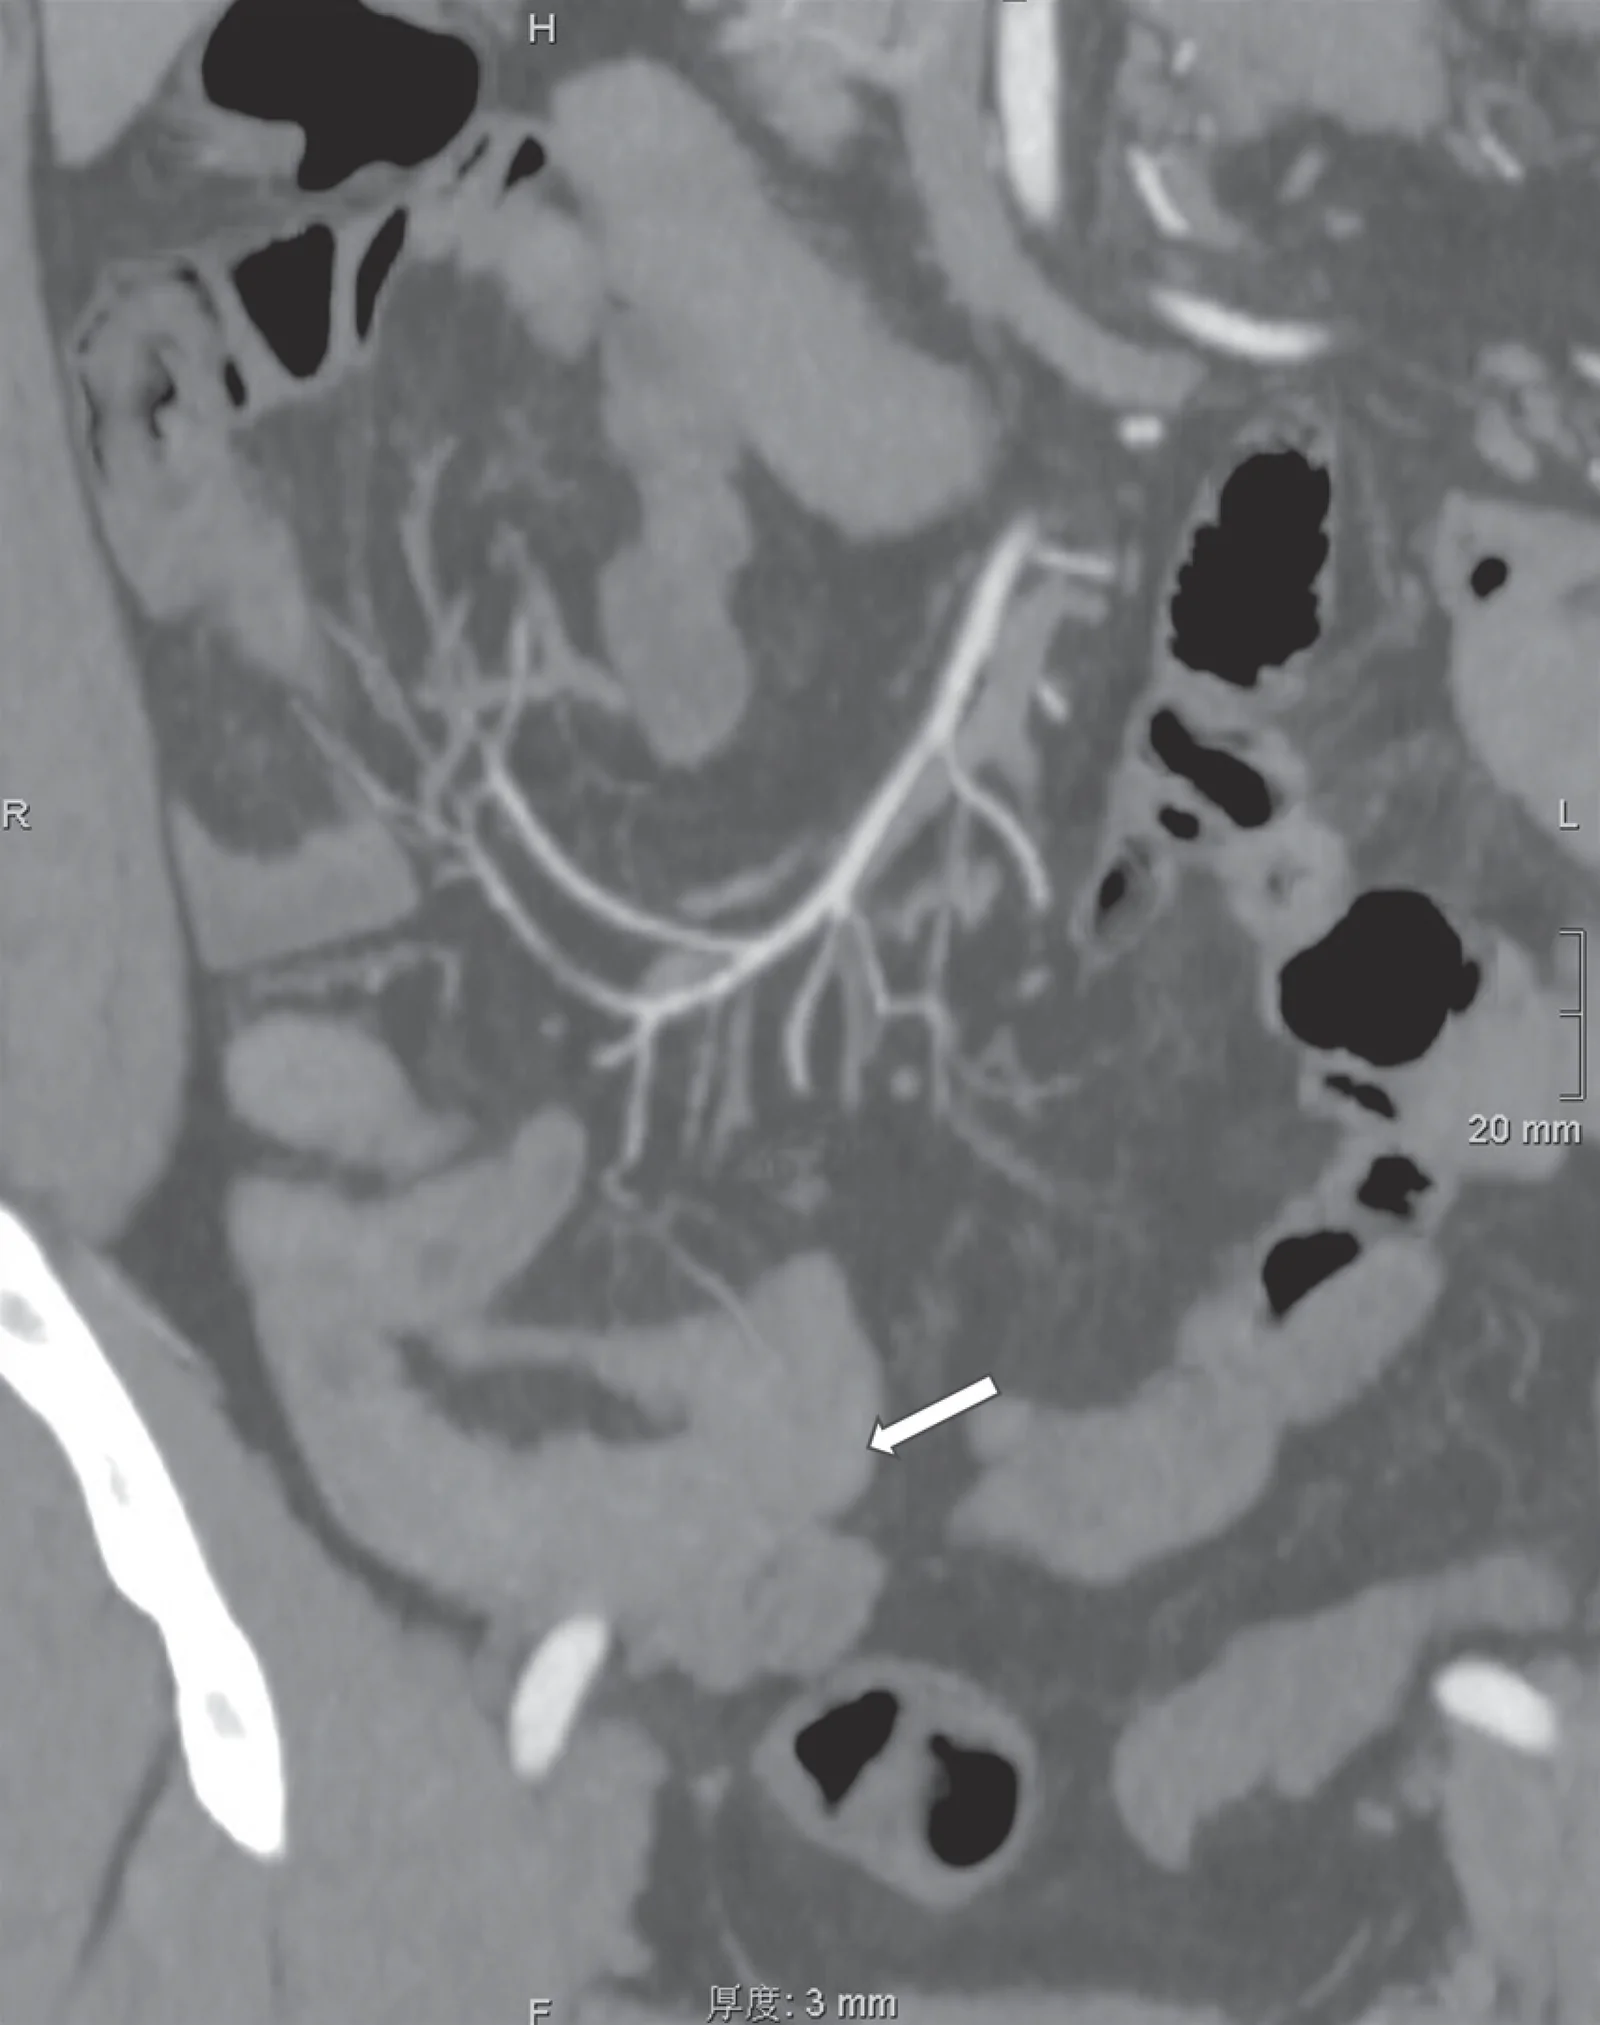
A computed tomography (CT) image showing a strip-like soft
